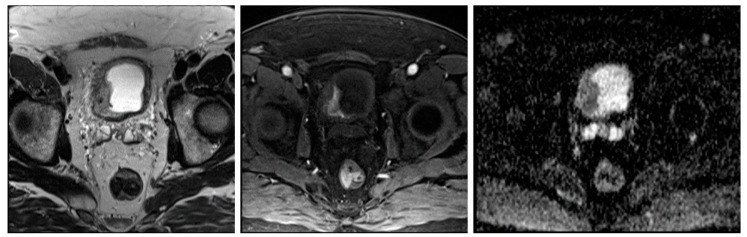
A bladder cancer case is depicted. An axial T2-weighted MRI shows an abnormal signal in the bladder (left). Post-contrast image reveals focal enhancement in the arterial phase (middle), while the ADC diffusion map indicates a low signal consistent with a high-grade tumor (right).
